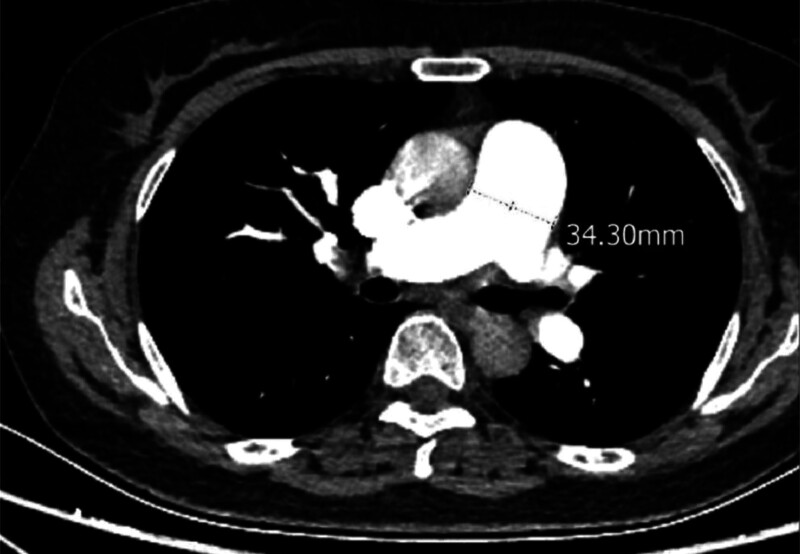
CT angiography of pulmonary arteries revealed the diameter of the pulmonary artery was 34.30 mm.
